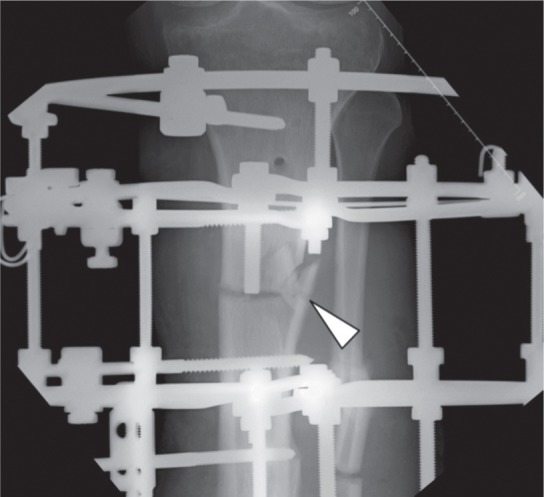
Radiograph showing Type III corticotomy with a “butterfly” fragment.
